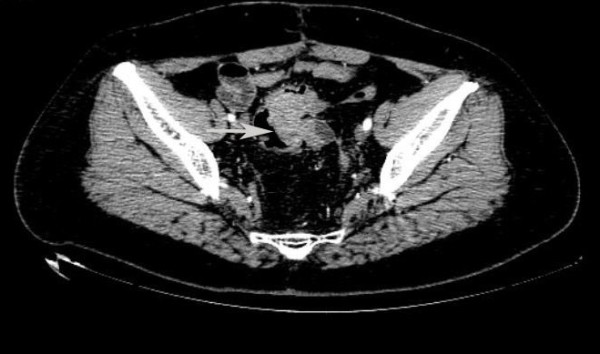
Contrast-enhanced CT showed eccentric wall thickening of the distal sigmoid colon with a significantly enhanced soft tissue density mass causing an apparent stenosis (arrow) and nodular low density shadow in the left side. CT, computed tomography.
